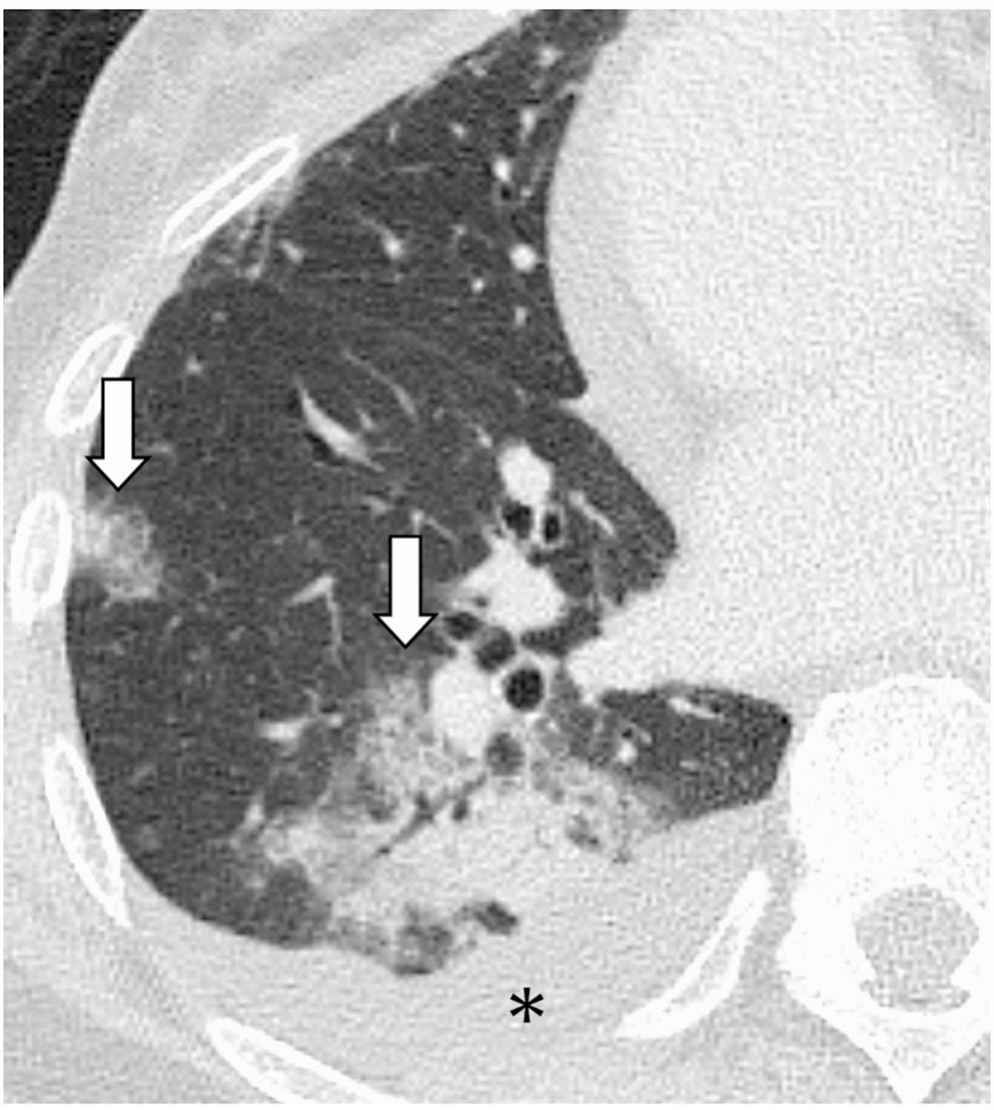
Abemaciclib-related pneumonitis in a 76-year-old woman. The patient, who had multiple pulmonary, pleural, and bone metastases from luminal type breast cancer, was receiving letrozole and abemaciclib. High-resolution CT (HRCT) image shows multiple ground-glass opacities and consolidation in the right lower lobe of the lung (arrows). Drug-associated interstitial lung disease with organizing pneumonia (OP) pattern due to abemaciclib was suspected. After discontinuation of abemaciclib, the opacities subsequently improved. Right pleural effusion (*) is also seen
